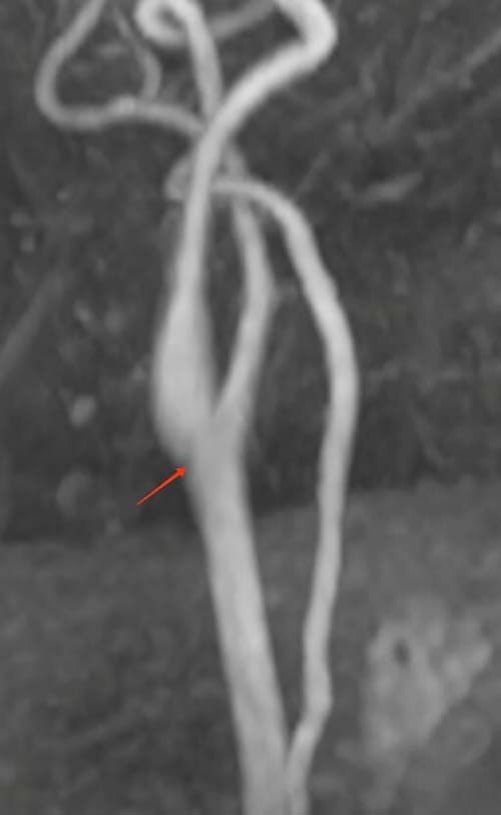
MRI reconstruction image of the left carotid bifurcation showing the carotid diaphragm.
